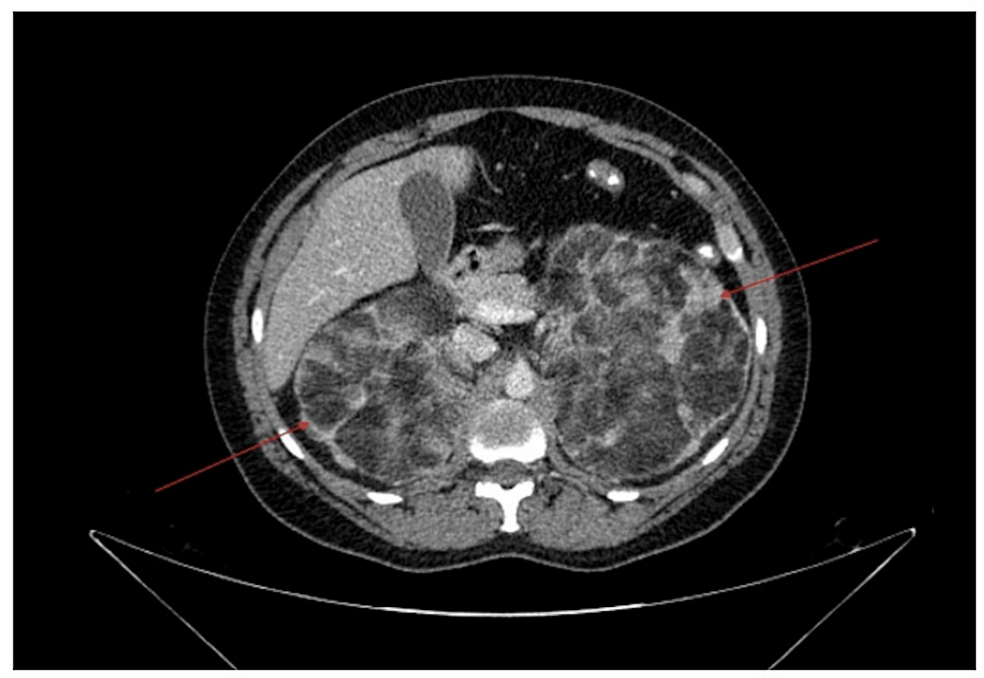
Cross section of CT abdomen and pelvis with contrast. Bilateral fat-containing adrenal masses characteristic of adrenal myelolipomas (red arrows) are visible. CT: computed tomography
